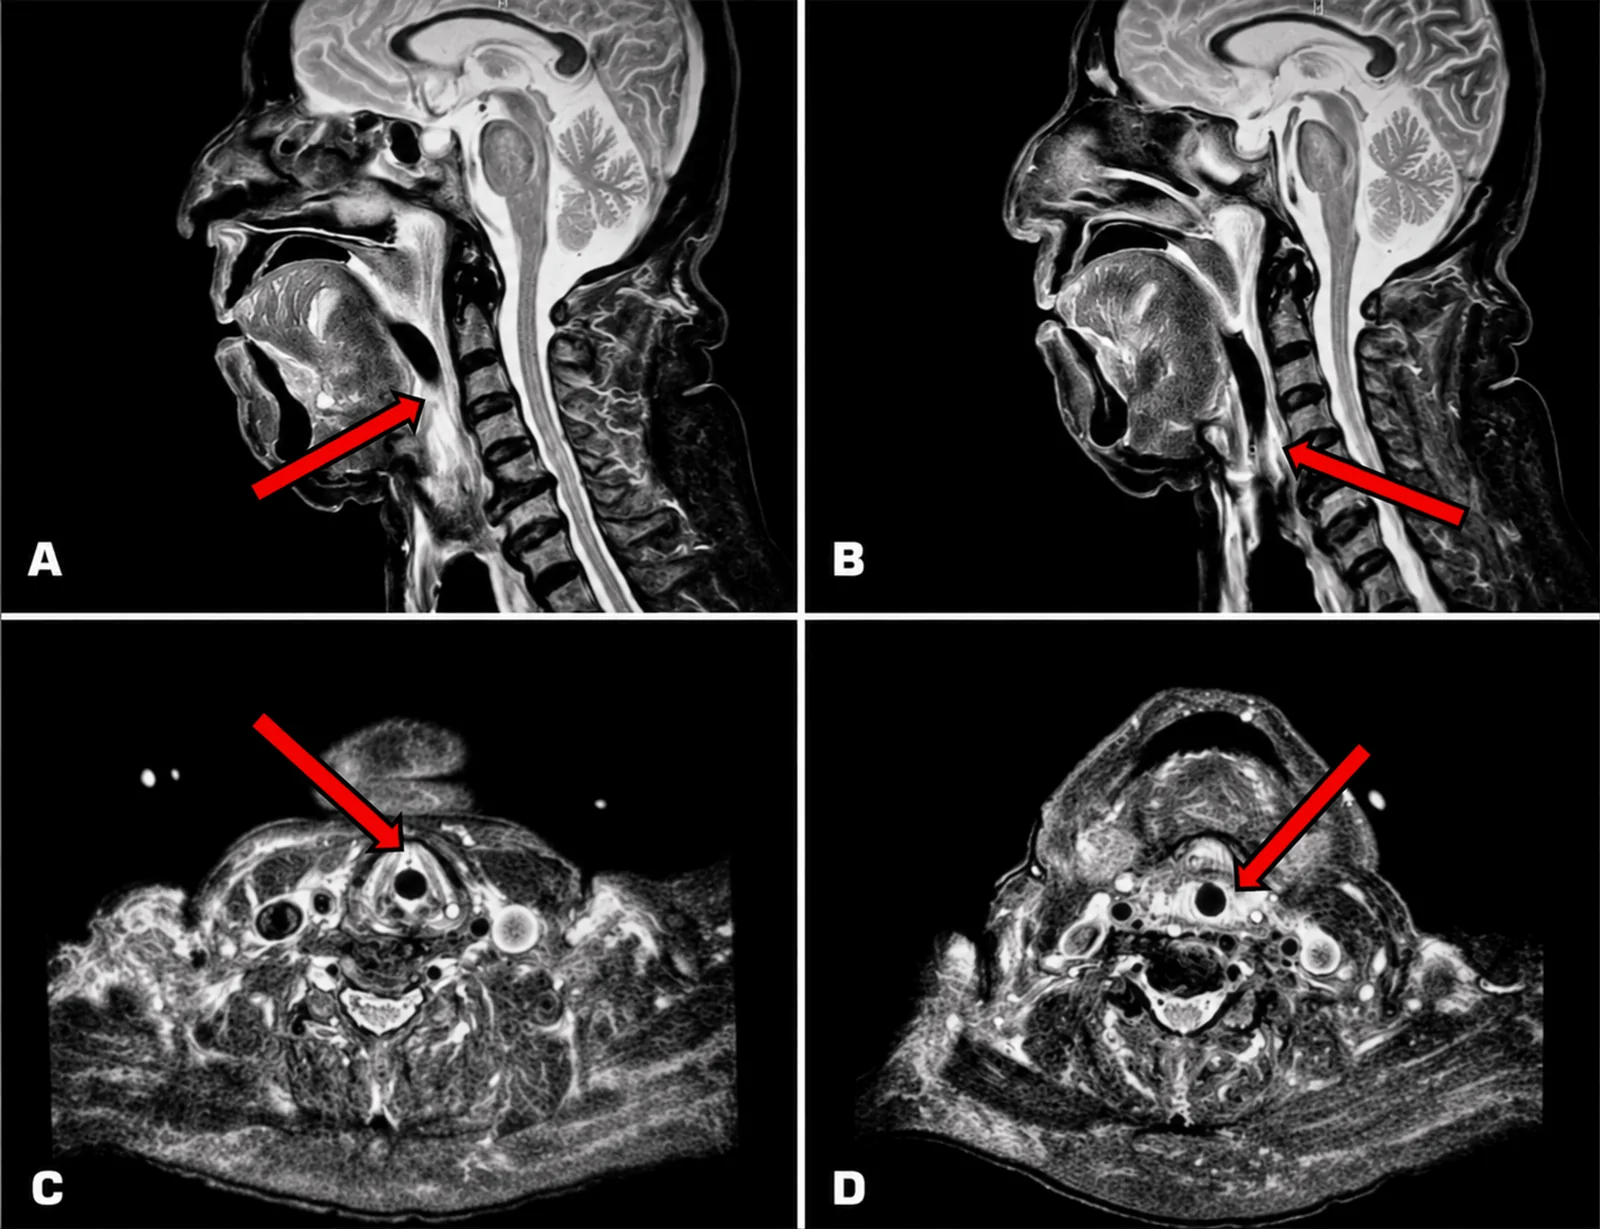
Magnetic resonance imaging of the brain and cervical region showed upper-airway edema with no evidence of acute ischemic stroke. (A, B) Sagittal T2-weighted images demonstrating diffuse upper-airway edema along the posterior pharyngeal wall and supraglottic vestibule (arrows). (C, D) Axial T2-weighted images at the level of the upper larynx showing circumferential edema of the aryepiglottic folds and pre-epiglottic fat spaces (arrows), resulting in critical narrowing of the residual airway passage.
